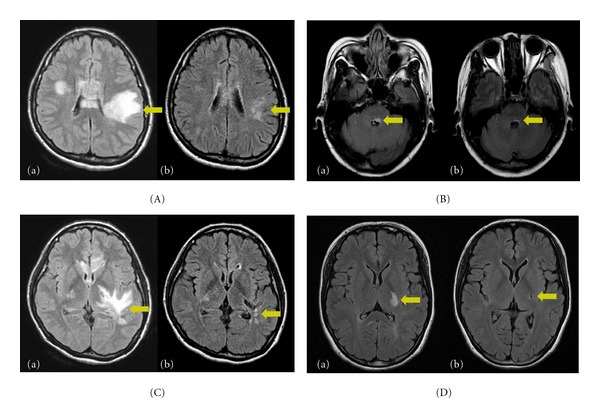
MRI findings at acute manifestation and after symptoms resolved. Fluid-attenuated inversion recovery (FLAIR) abnormalities in a patient with encephalopathy (A-a) and T2 signal abnormalities in a patient with brainstem sign (B-a) almost resolved over a few months (A-b, B-b). However, the lesions involving the corticospinal tracts, especially the posterior limb of the internal capsule (C-a, D-a) showed cavitary changes on followup MRIs (C-b, D-b) [9].
